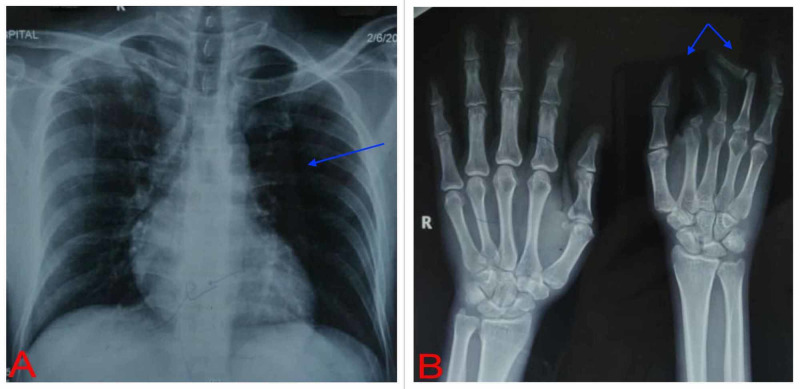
Radiographs (A) Chest X-ray showing hyperlucency on the left side with normal lung parenchyma, heart shadow, and ribs; (B) X-ray of the left hand showing hypoplasia of the proximal phalanx, aplasia of the middle and distal phalanges of the 2nd digit, and aplasia of the middle phalanges of the 3rd and 4th digits.
